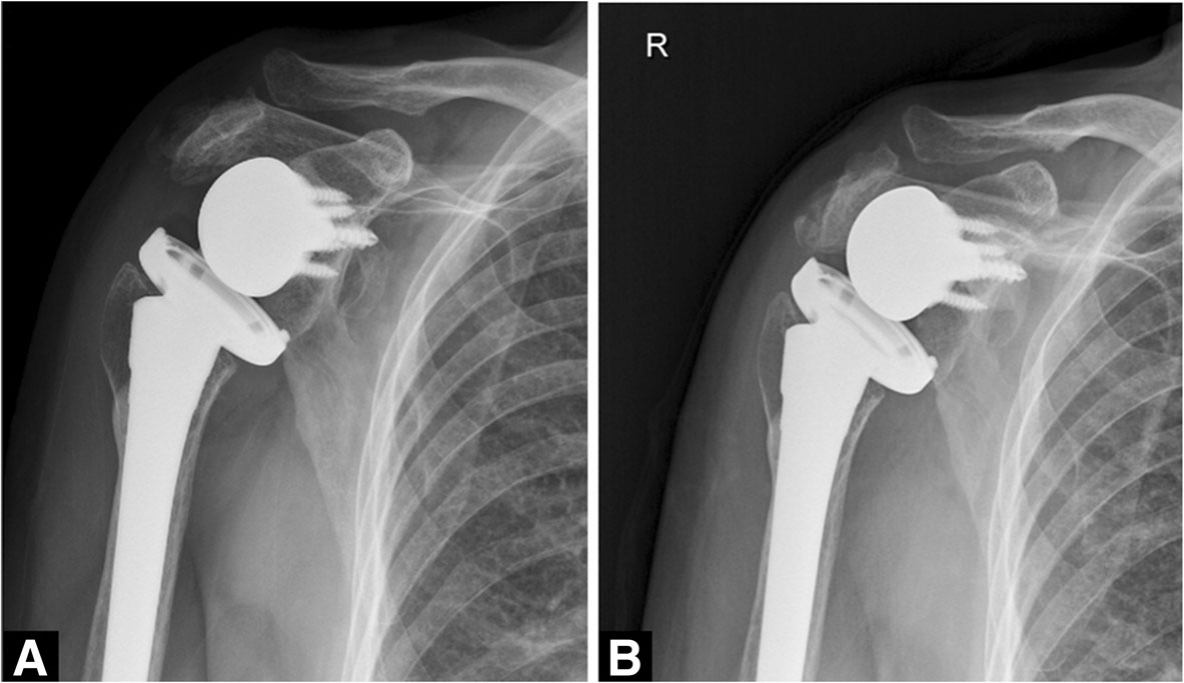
A 79-year-old female patient with acromial fracture. a Postoperative anteroposterior radiograph of the shoulder at 18 months follow-up. b Anteroposterior radiograph at 22 months follow-up after conservative treatment shows union of the acromion
